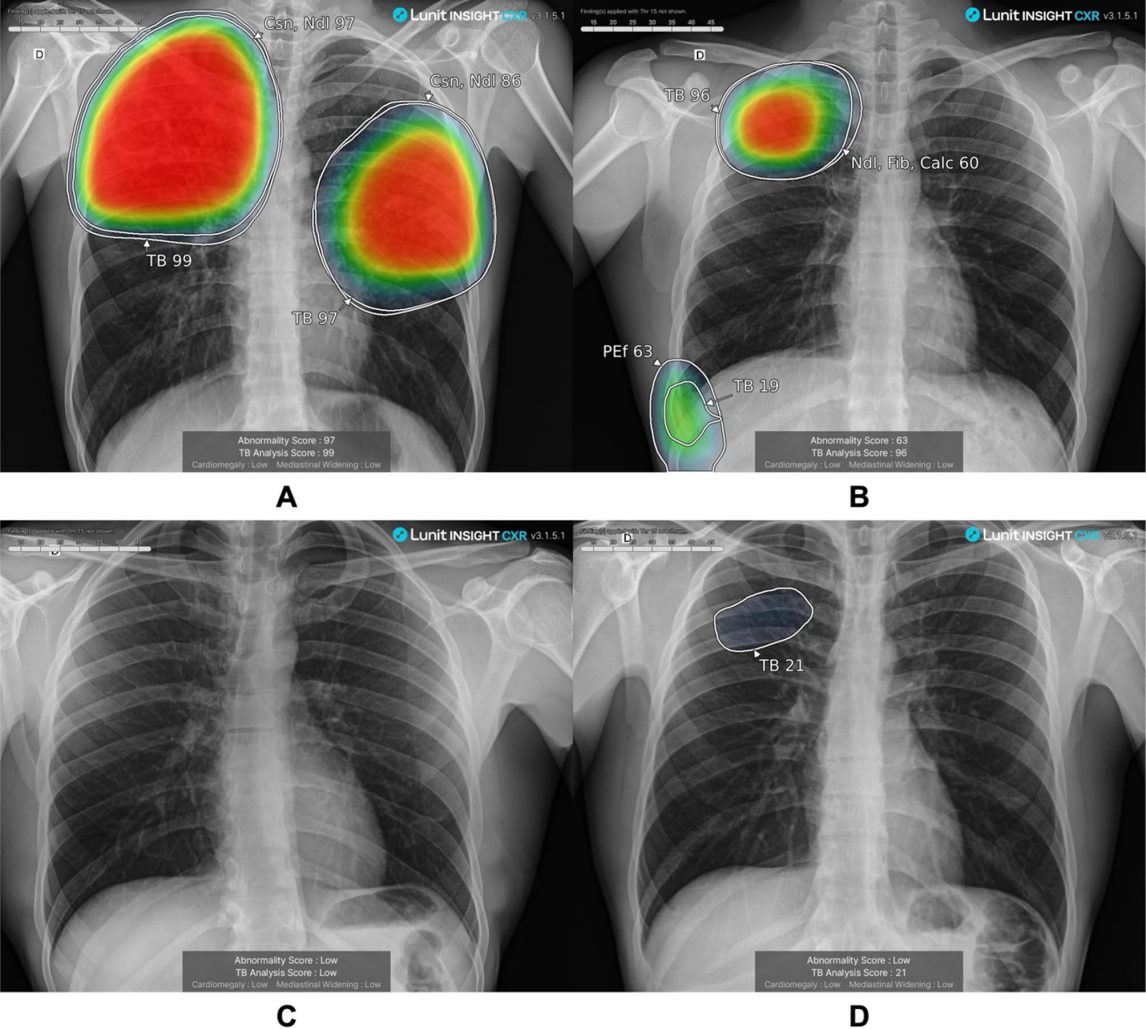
Chest X-ray images produced with FDR Xair and scored with LunitTB for two individuals with tuberculosis (A and B) and two individuals without tuberculosis (C and D)
